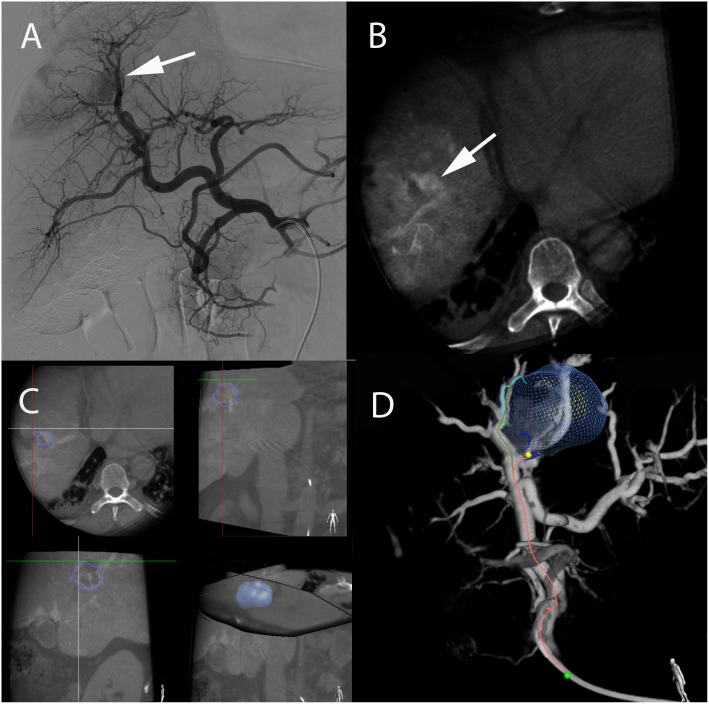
Real-time tumor tracking and detection of HCC feeder in a 57-year-old male with hepatitis B and alcoholic cirrhosis. (A) Angiography from the celiac axis showing an area of blush corresponding to the target tumor (arrow) and multiple vessel superposition of putative tumor-feeding arteries. This complex arterial anatomy made tumor feeders very difficult to identify. (B) Intraprocedural contrast-enhanced CBCT performed with soluble contrast medium injection through the microcatheter in the proper hepatic artery depicted a nodular hyperattenuating area corresponding to the tumor (white arrow). (C) Based on that CBCT, the tumor could be precisely segmented in 3D and a 3D reconstruction of the arterial anatomy of the liver could be obtained. (D) A tumor-feeding vessels detection software (EmboGuide, Philips) could then be used which displayed the target lesion together with 3D reconstructed angiogram. The microcatheter tip (green dot), tumor feeding vessel (in red) and its branches (green, light and dark blue) are shown. This superimposed 3D target tumor with tumor feeders can be superimposed to real-time fluoroscopy (not shown) allowing for optimal superselective microcatheter placement before treatment administration.
